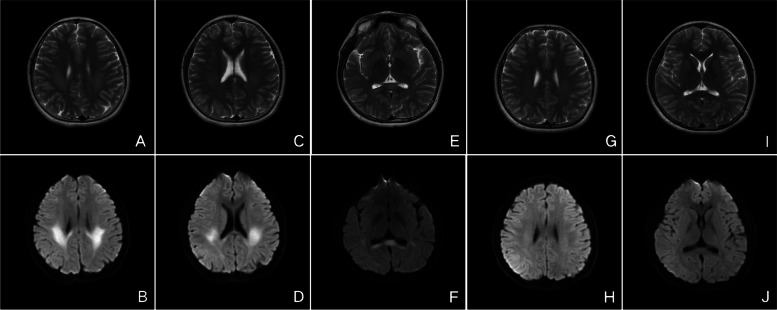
Cranial MRI of case 2 in 2017. Initial cranial MRI showed lesions in the entire corpus callosum and diffuse white matter T2-weighted images (A,B,E) and marked hyperintensity on DWI (B,D,F). Follow-up MRI showed resolution of the lesions on T2 (G,I) and DWI (H,J) after 3 months
